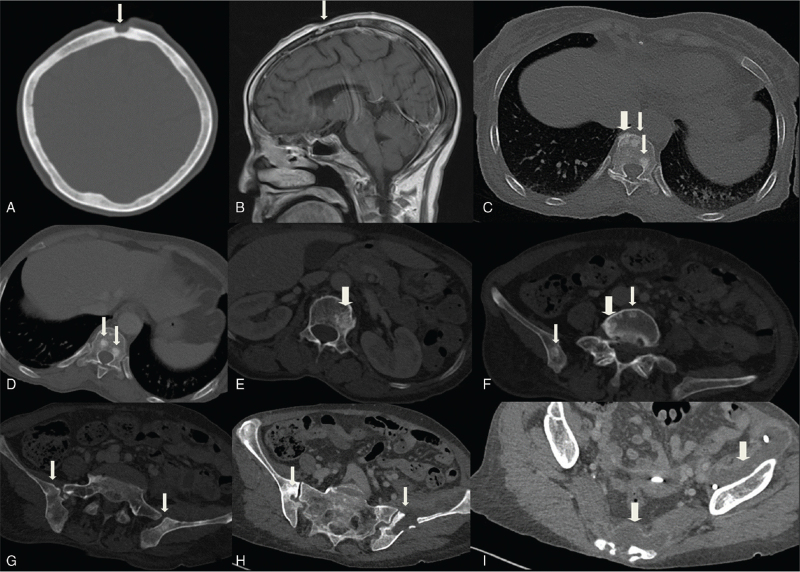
(A) Soft tissue defect of the forehead with local destruction of the frontal bone (arrow). (B) Sagittal T1-weighted imaging with enhancement shows plaque enhancement of the destruction of the skull (arrow). (C-G) Multiple sclerotic lesions were found in the vertebral bodies and both sides of the iliac bones that presented as nodules or clumps of high-density shadows (straight arrows), and lesions at the edge of the vertebral body showed cortical sclerosis and irregularity (thick straight arrows). (F) Internal low-density shadows can be seen in part of the lesion (thin straight arrows). (H-I) Both sacroiliac joints showed bony destruction and abscess formation in front of the left iliac bone and sacrum (thick straight arrows; a drainage tube can be seen inside the abscess).
